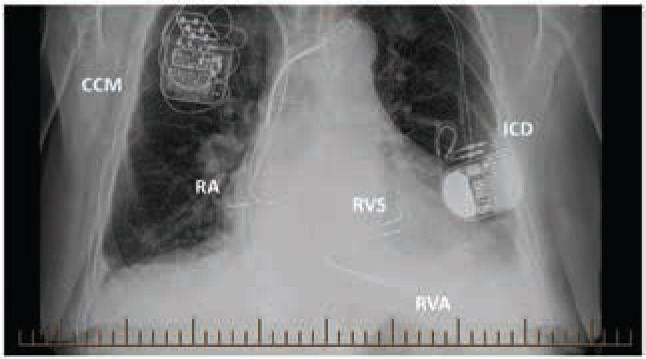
Anteroposterior chest X-ray of a patient with an implanted CCM device and a dual-chamber ICD. CCM: cardiac contractility modulator generator; ICD: implantable defibrillator generator; RVS: CCM ventricular leads placed in the right ventricular septum; RVA: RV apical ICD lead; RA: right atrial ICD and CCM leads.
